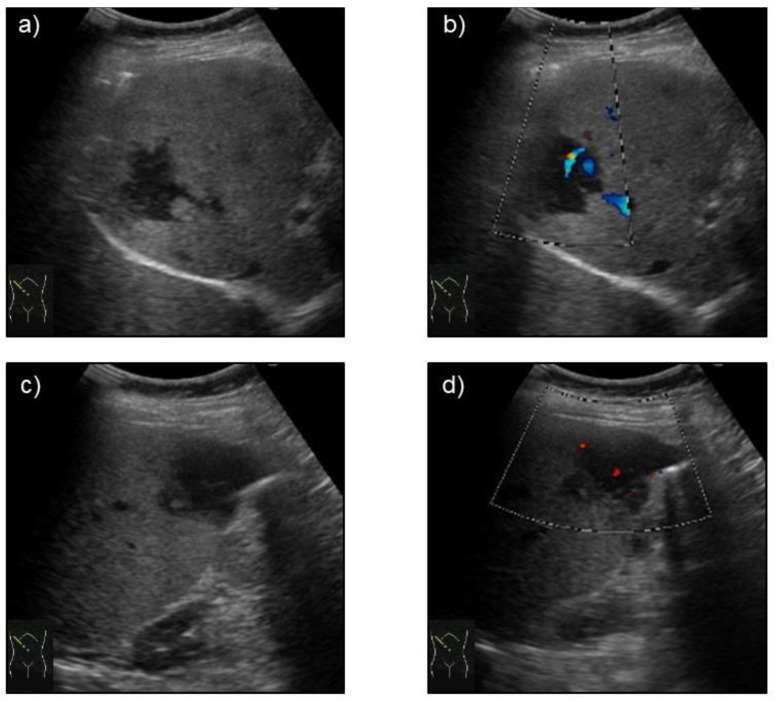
B-mode ultrasonography depicted a hypo-echoic tumor lesion at segment S8 in the liver (a), through which a penetrating vessel was visualized by color Doppler imaging (b). B-mode ultrasonography showed a hypo-echoic tumor lesion at segment S5 in the liver (c), through which a penetrating vessel was visualized by color Doppler imaging (d).
